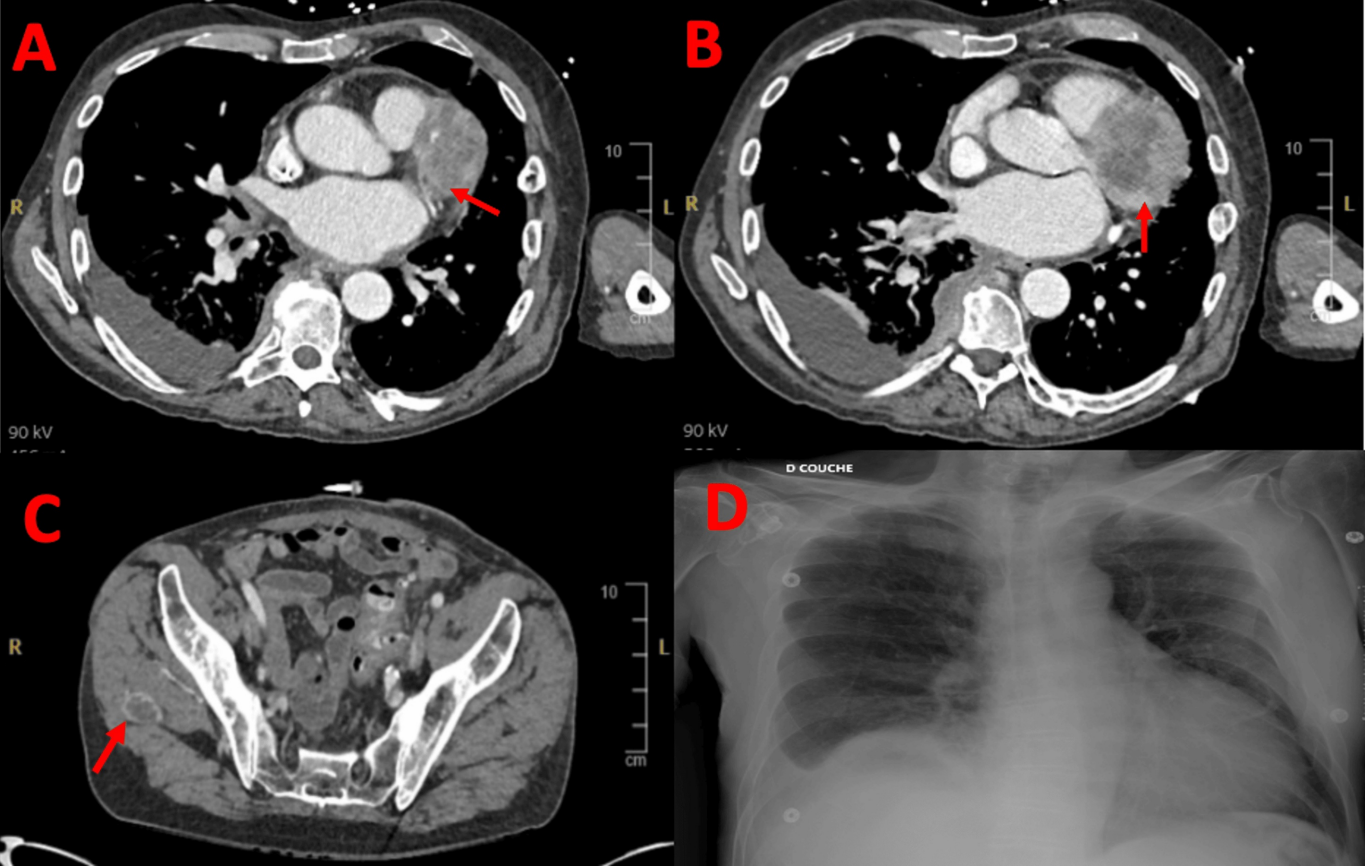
Other imaging modalities. A+B: CT scan images showing the cardiac mass (red arrows). C: CT scan image showing gluteal muscle metastasis. D: Chest X-ray that does not show any specific finding.
